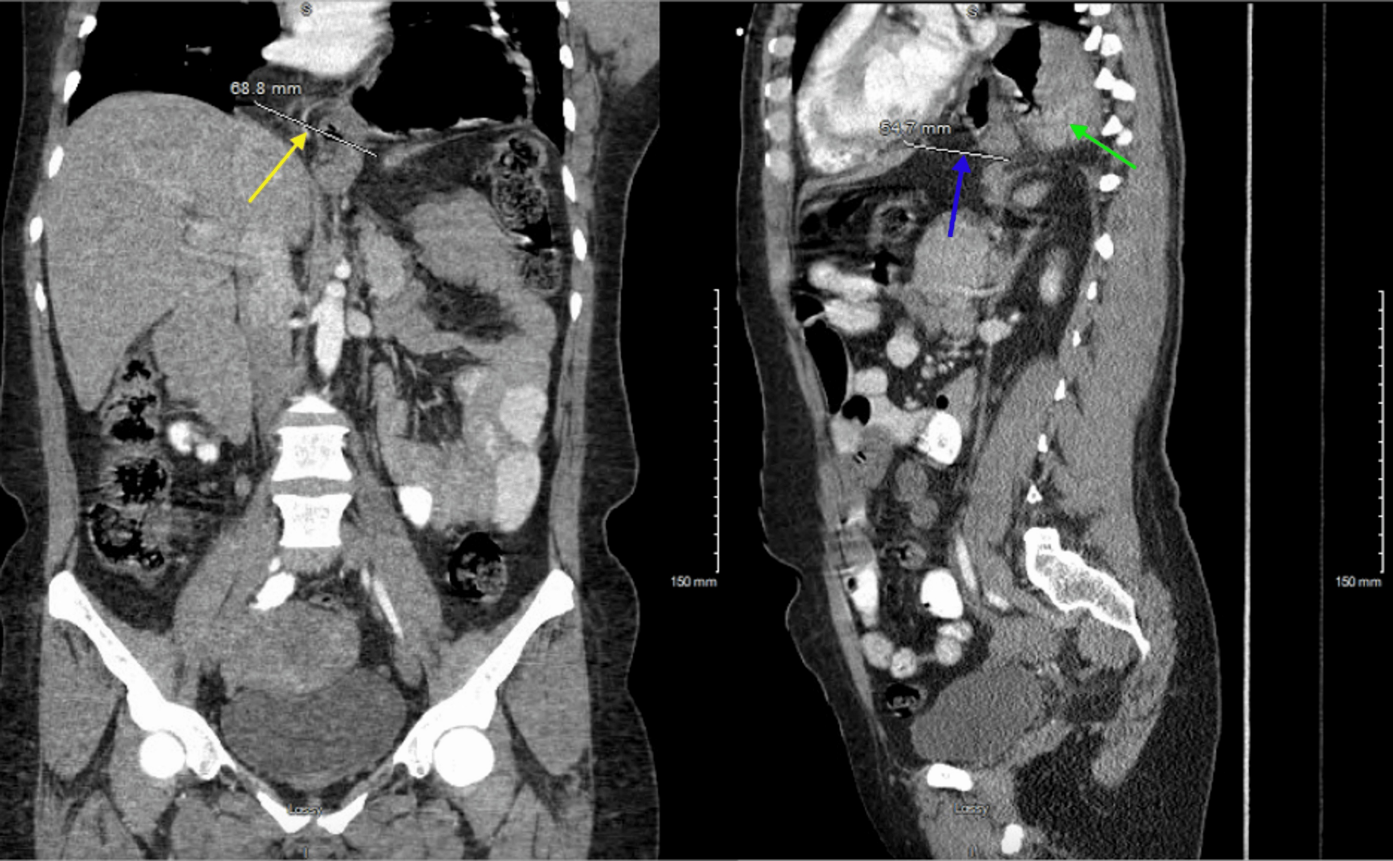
CT scan of the abdomen and pelvis shows a large diaphragmatic hernia at the hiatus. In a coronal section, the defect in the diaphragm is 7 cm in diameter, medial to lateral (yellow arrow), and in a sagıttal cross section, it is 5 cm in diameter, anterior to posterior (blue arrow). Most of the stomach is intrathoracic, with the antrum and distal stomach lying directly anterior to the fundus in a paraform (green arrow)
